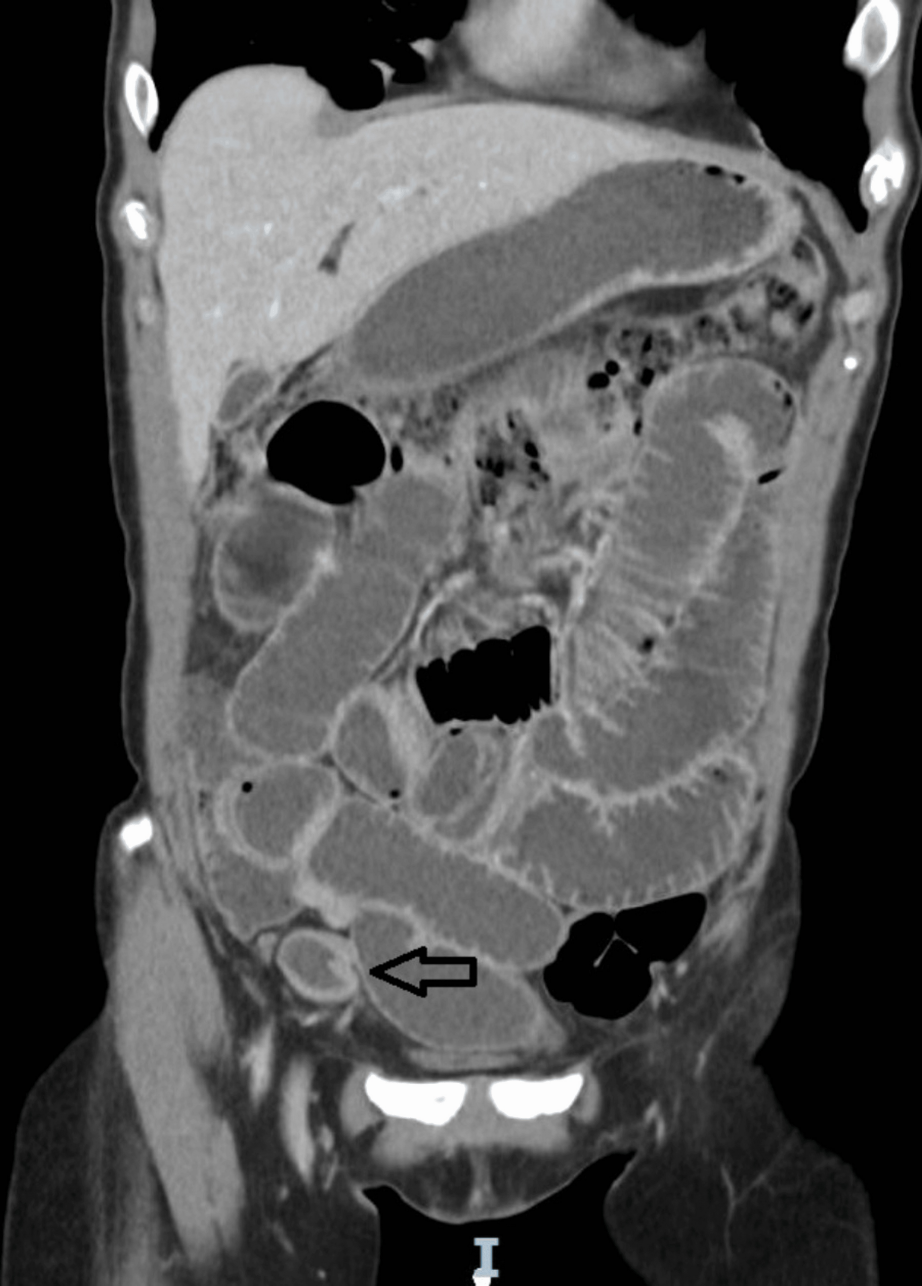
CT image showing that the patient had mechanical intestinal obstruction
